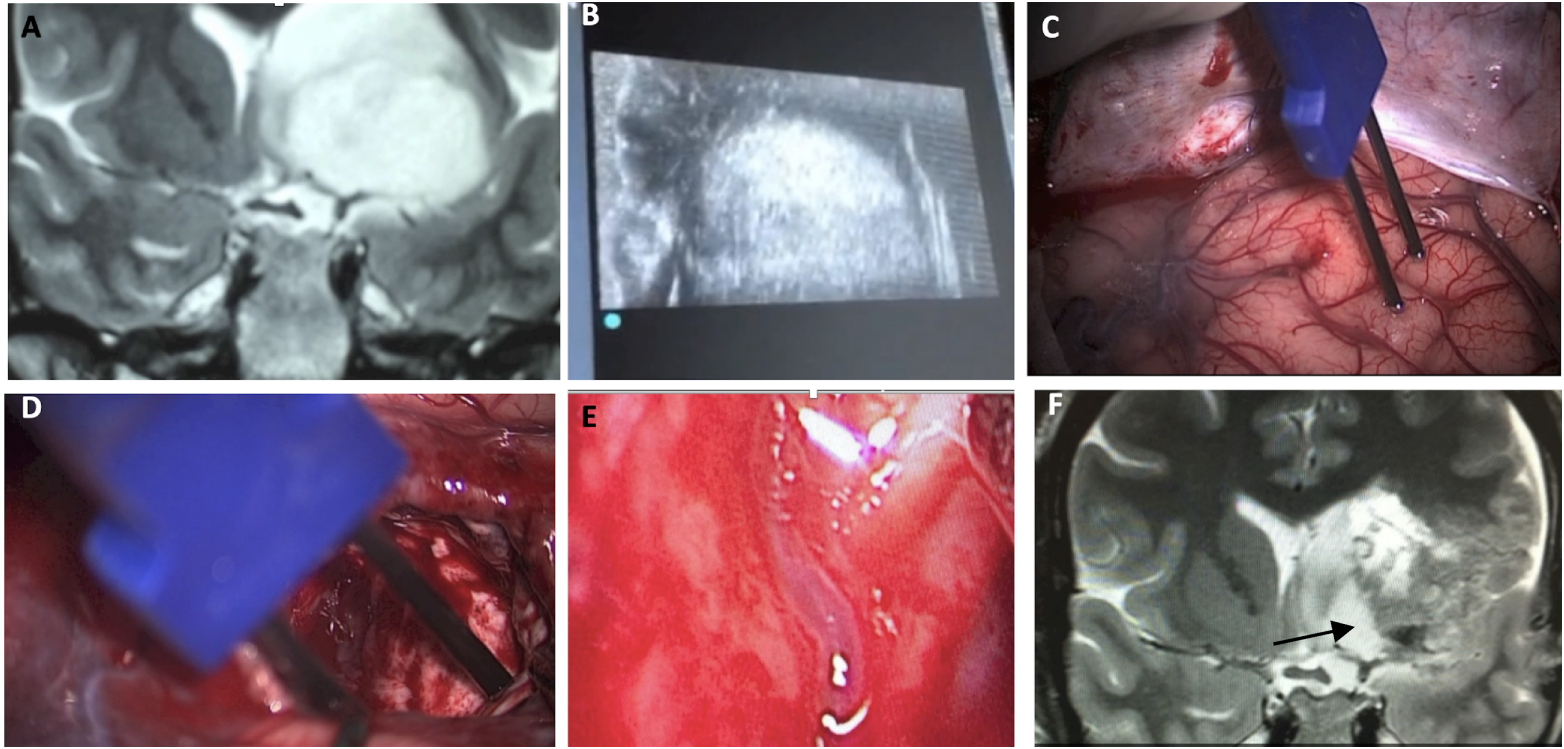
A microsurgical resection of a left insular glioma with LSTa encasement. (A) Coronal T2-weighted MRI showing left-sided insular glioma suggesting crossing over the Porto Alegre indicating high probability of LSTa tumoral encasement; (B) intraoperative echography; (C) cortical brain mapping was silent on the so called “Braca's area”; (D) subcortical brain (day 1) showing residual tumor medial to the Porto Alegre line, as it was the medial resection limit. (E) Intraoperative view of the LSTa. (F) Part of the tumor medial to the Porto Alegre line was not resected (arrow). Figure from Isolan et al. (36).
